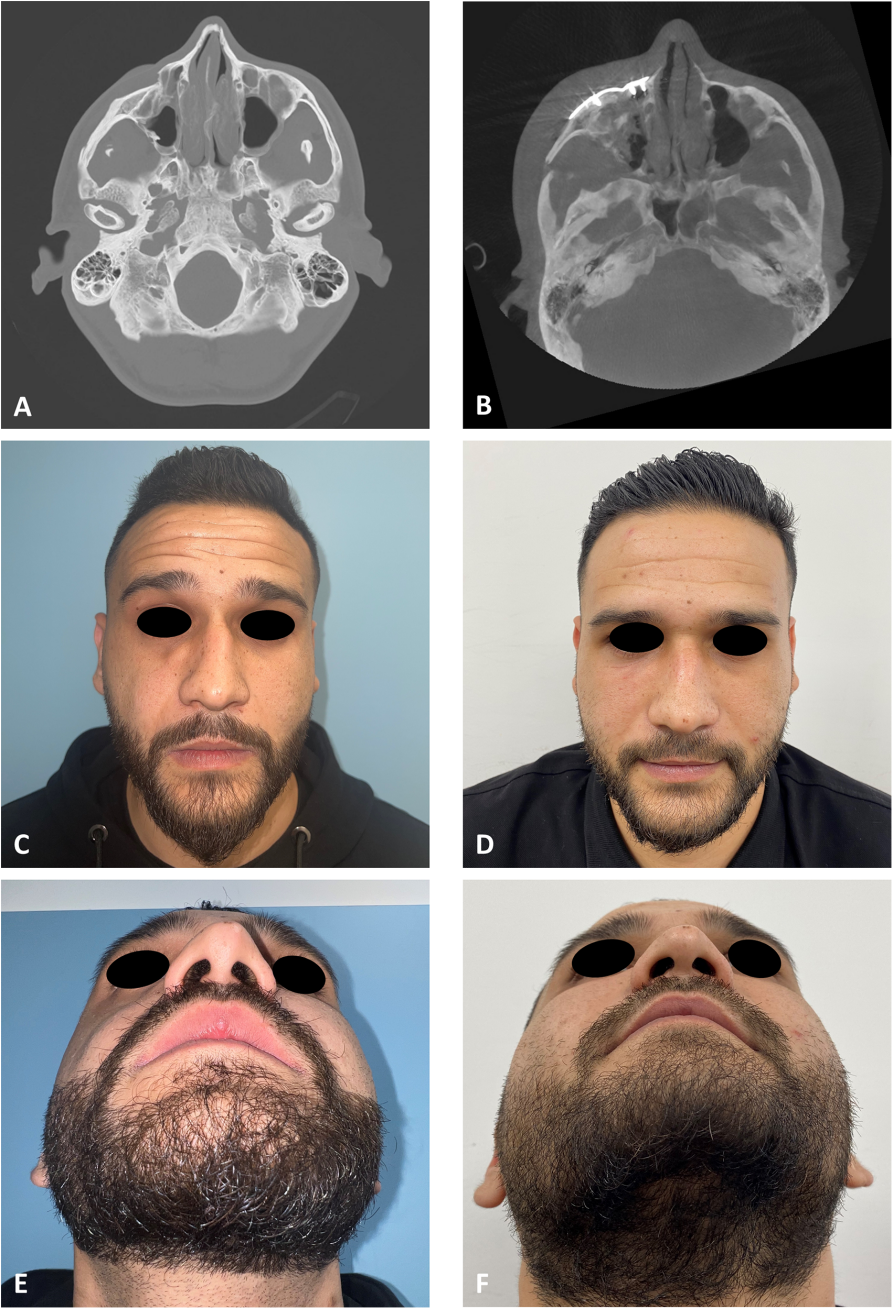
Clinical and radiological results. (A) CT stacking of a preoperative axial view demonstrating a depressed right zygoma. (B) CT stacking of a postoperative axial view demonstrating a leveled right zygoma. (C) Clinical preoperative en face view emphasizing the step deformity in the infraorbital rim. (D) Clinical postoperative en face view. (E) Clinical preoperative worm view emphasizing the depressed right zygoma. (F) Clinical postoperative worm view.
